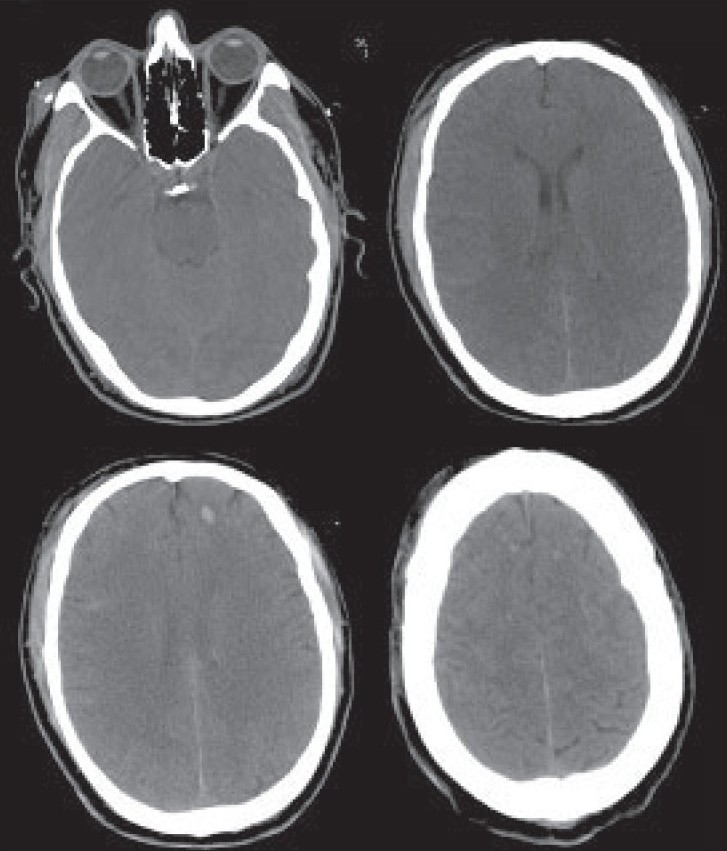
Brain CT scan showing few small cortical contusions. There are no mass lesions or midline shift; the ventricles and perimesencephalic cisterns are normal
